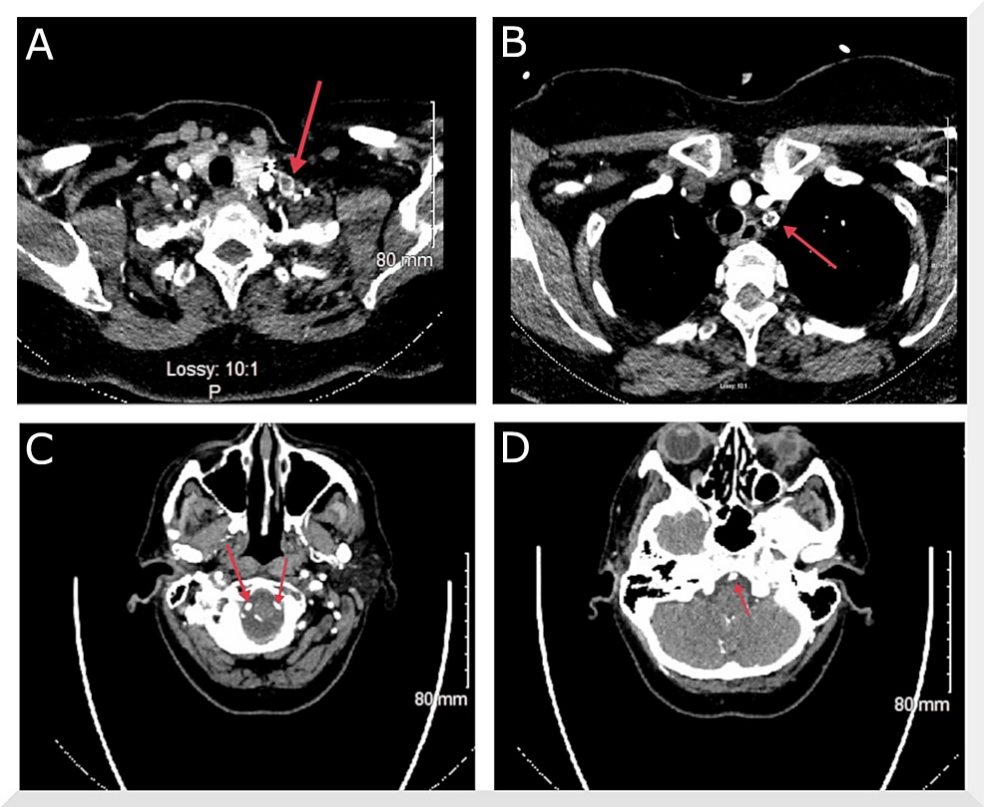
CTA of the head and neck. A) Occlusion of the left common carotid-subclavian artery bypass graft. B) Occlusion of the left subclavian artery stent. C) Patency of the vertebral arteries bilaterally. D) Patency of the basilar artery.
